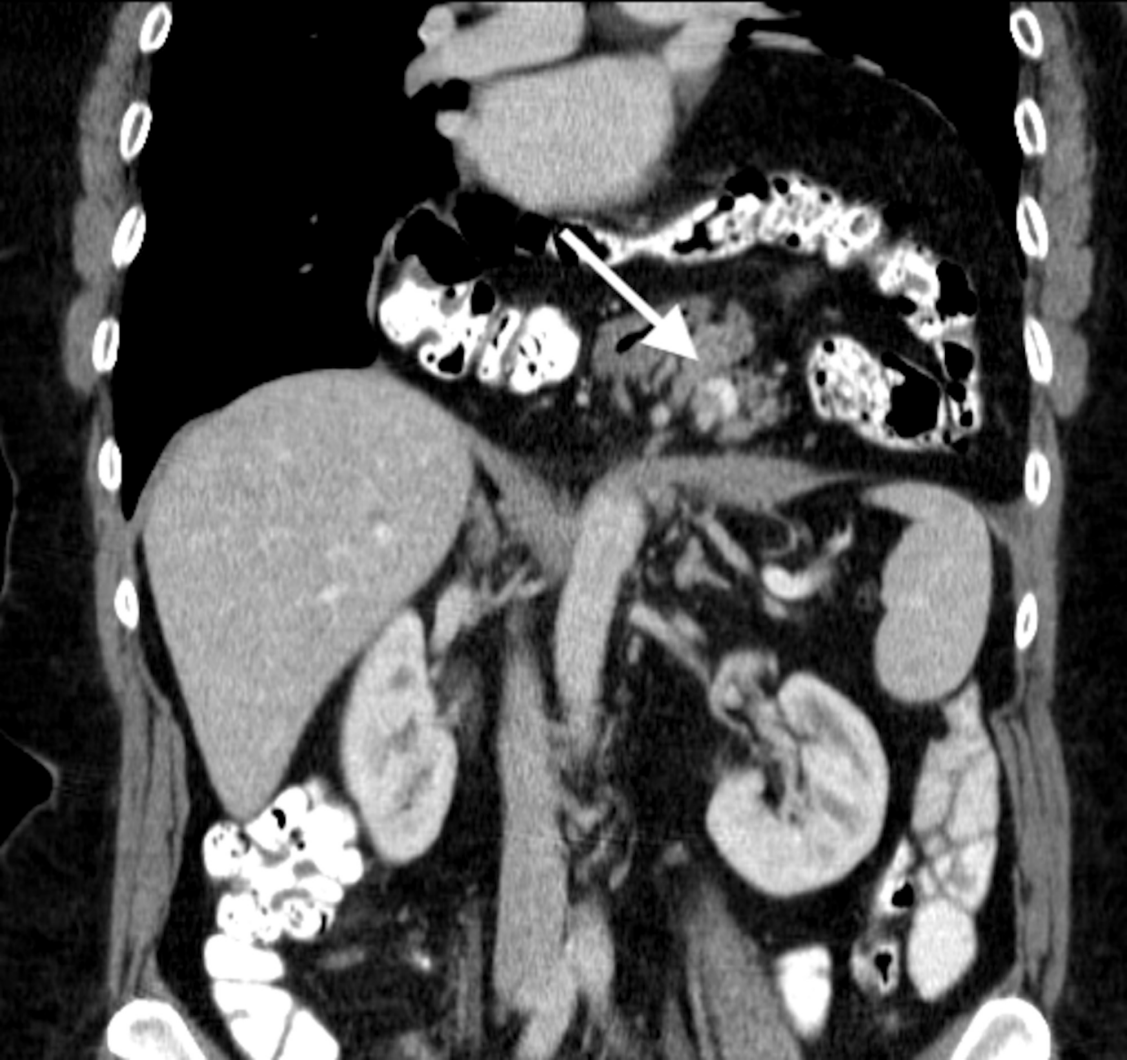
CT-scan of Patient B depicting herniation of the pancreas into the thoracic cavity.
